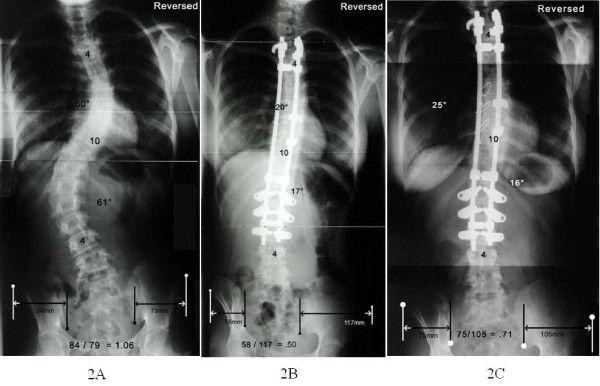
Standing posterior-anterior radiographs of a patient with a type 3 curve. The patient's postoperative TPPR increase did not resolve: preoperative (Fig. 2-A), postoperative (Fig. 2-B), and at 2 years, 3 months postoperative (Fig. 2-C). Patient has left thoracic-right thoracolumbar curve apexes, so the radiographs were viewed inverted horizontally (reversed).
